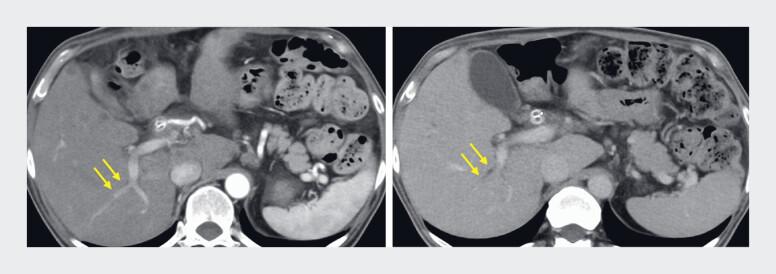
Follow-up CT obtained 49 days later during elevation of liver enzymes, demonstrating slight dilatation of bile duct limited to the posterior segment (arrow). Left: CT at the time of SBS MH-SEMS placement. Right: CT at 49 days after MH-SEMS placement. CT, computed tomography; MH-SEMS, multi-hole self-expandable metallic stent.
